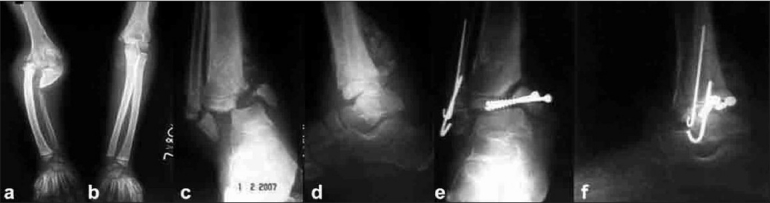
X-ray of both bones forearm with elbow joint (anteroposterior views) (a, b) showing atrophic nonunion of proximal ulna with displaced radial head on right side. X-ray of the right ankle (anteroposterior and lateral views) shows nonunion (c, d), of bimalleolar fracture and internal fixation and for nounion fracture dislocation of right ankle (e,f)
